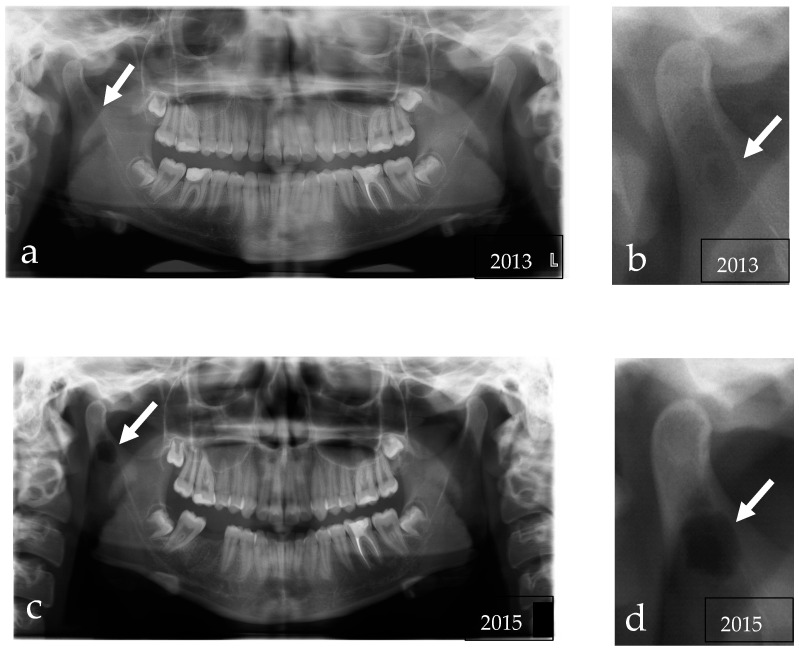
(a) The Rx Orthopantomography made in 2013 showing a diffuse, unilocular, and radiolucent lesion, with regular margins, located within condylar process; (b) Close view of the lesion; (c) The panoramic radiograph made in 2015 showing a small unilocular osteolytic lesion at the base of the right condylar process; (d) Close view of the lesion in the panoramic radiograph.
